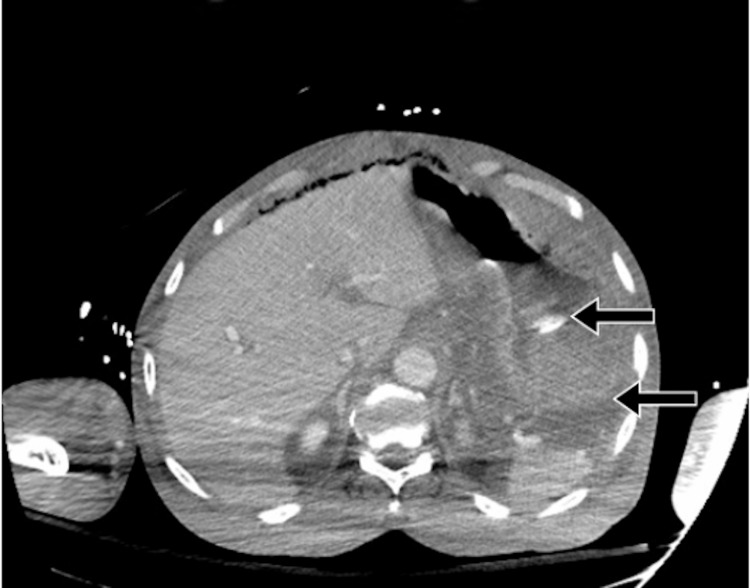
Abdomen/pelvis CT revealing hyperdense fluid in the stomach and OG tube in place, consistent with upper GI bleed (arrows).
